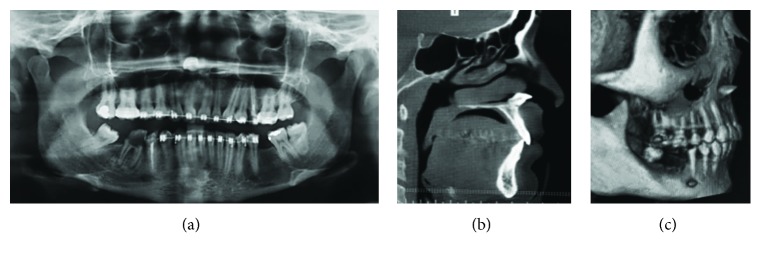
(a) Panoramic radiograph shows an ectopic tooth in the nasal cavity. (b) Sagittal cut of CT scan showing the horizontal orientation of the right superior incisor tooth inside the nasal nostril. (c) 3D reconstruction of CT scan showing the ectopic position of element 11.
